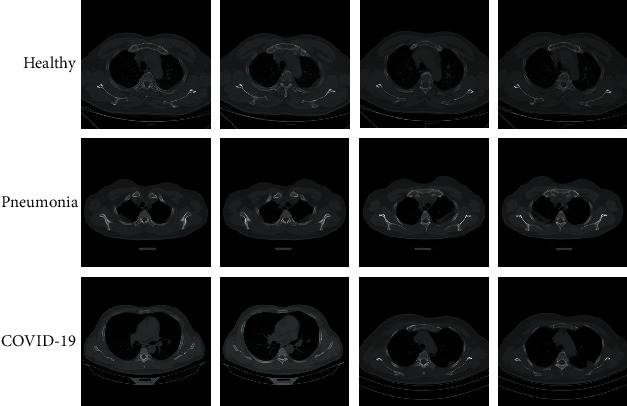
Some examples of the CT scan images collected from the MosMedData dataset [25].
